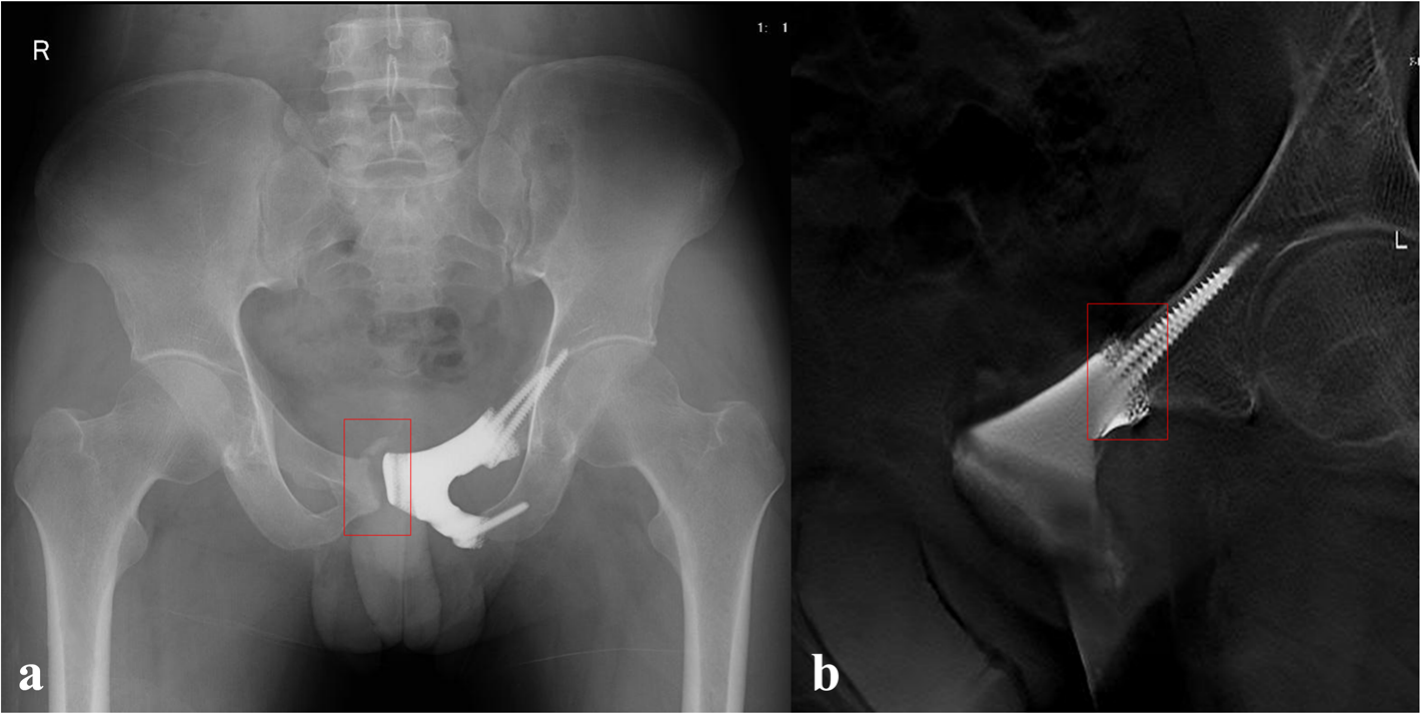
Four months after the operation, X-ray showed bone wear on the normal side pubis (a); Six months after operation, T-SMART showed preliminary osseointegration (b)
